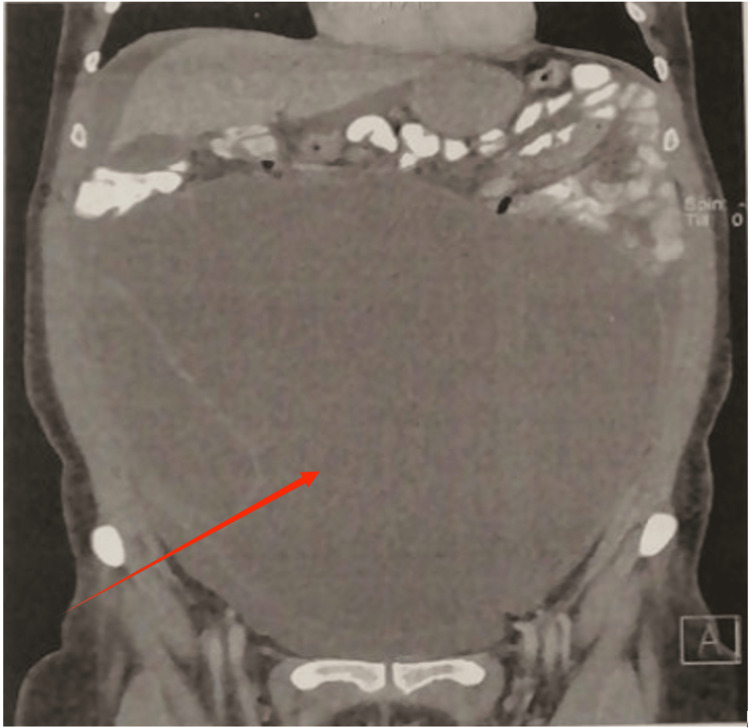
Coronal abdominal magnetic resonance imaging (MRI) revealed a large, near-water-density cystic mass with internal thick septations and mural calcification (red arrow).
